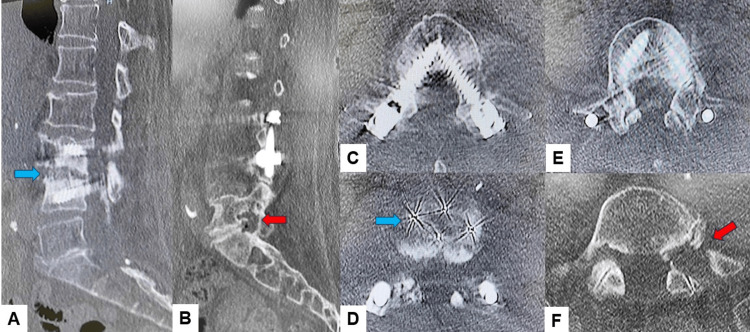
Final follow-up CT. A: Midsagittal reconstructed CT image; B: left parasagittal reconstructed CT image; C: axial CT image at L3; D: axial CT image at L3/4; E: axial CT image at L4; F: axial CT image at L5/S1. Blue arrows indicate the OLIF cage. The red arrow demonstrates adequate decompression of the left L5 exiting nerve root. Postoperatively, lumbar lordosis improved from 25° to 32°, and foraminal height increased from 6.8 mm to 9.4 mm, respectively. OLIF: Oblique lumbar interbody fusion.
